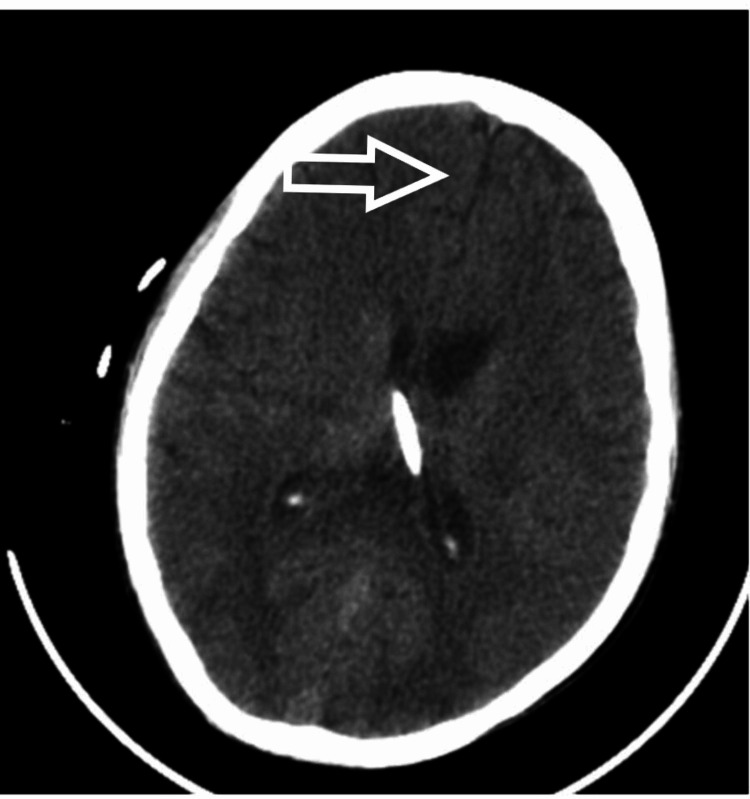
NCCT head showing hydrocephalus and midline shift - view 1 NCCT: noncontrast computed tomography
